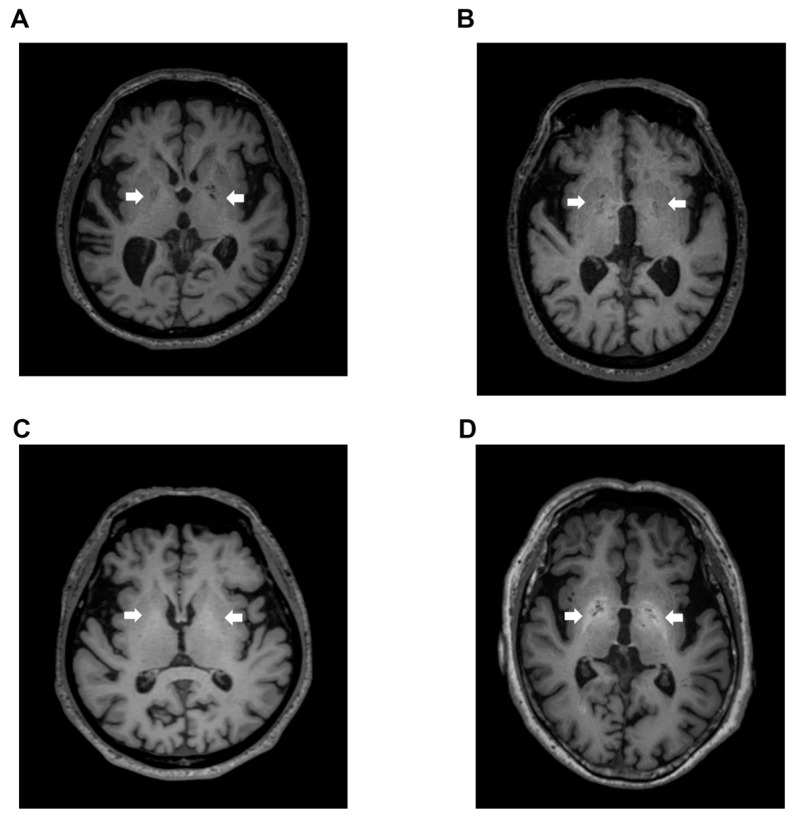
T1-weighted images. (A) Transverse T1-weighted imaging from a 74-year-old healthy subject. Signal intensity value of GP (arrow) was 0.952, which is below the cut-off value of 0.994; (B) Transverse T1-weighted imaging from a 79-year-old LC patient. Signal intensity value of GP (arrow) was 0.992, also below the cut-off value of 0.994; (C) Transverse T1-weighted imaging from a 63-year-old LC patient. Signal intensity value of GP (arrow) was 1.006, which exceeded the cut-off value of 0.994; (D) Transverse T1-weighted imaging from a 68-year-old HE patient. Signal intensity value of GP (arrow) was 1.024, also exceeding the cut-off value of 0.994. GP, globus pallidus; LC, liver cirrhosis; HE, hepatic encephalopathy.
